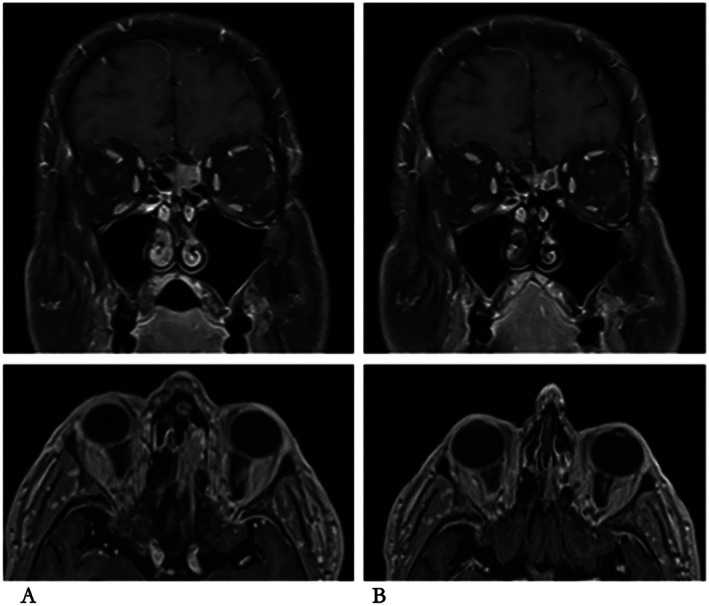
Ethmoidal and leptomeningeal involvement with lymphoplasmacytic lymphoma in Patient C. MRI head pre‐ and post‐Zanubrutinib treatment. (A) pre‐Zanubrutinib in coronal section (above), and axial section (below). (B) 3 months post start of Zanubrutinib in coronal section (above), and axial section (below).
